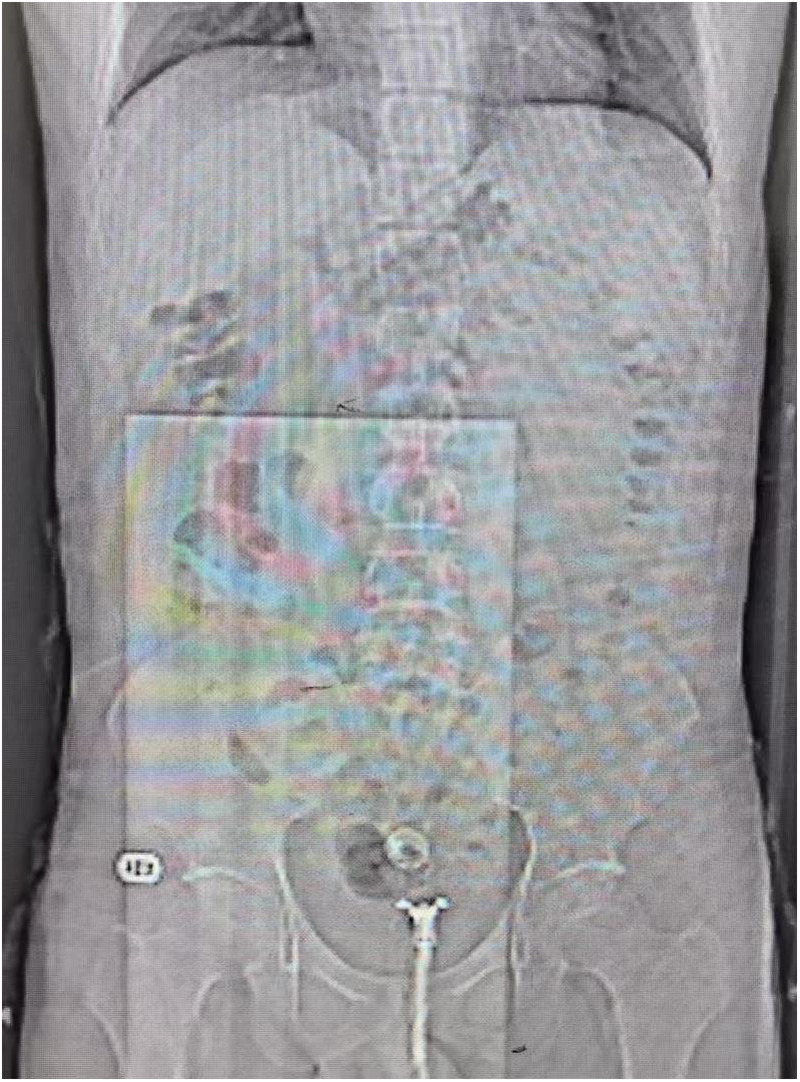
x-ray was initially performed but failed to clearly identify the foreign body or signs of perforation.
